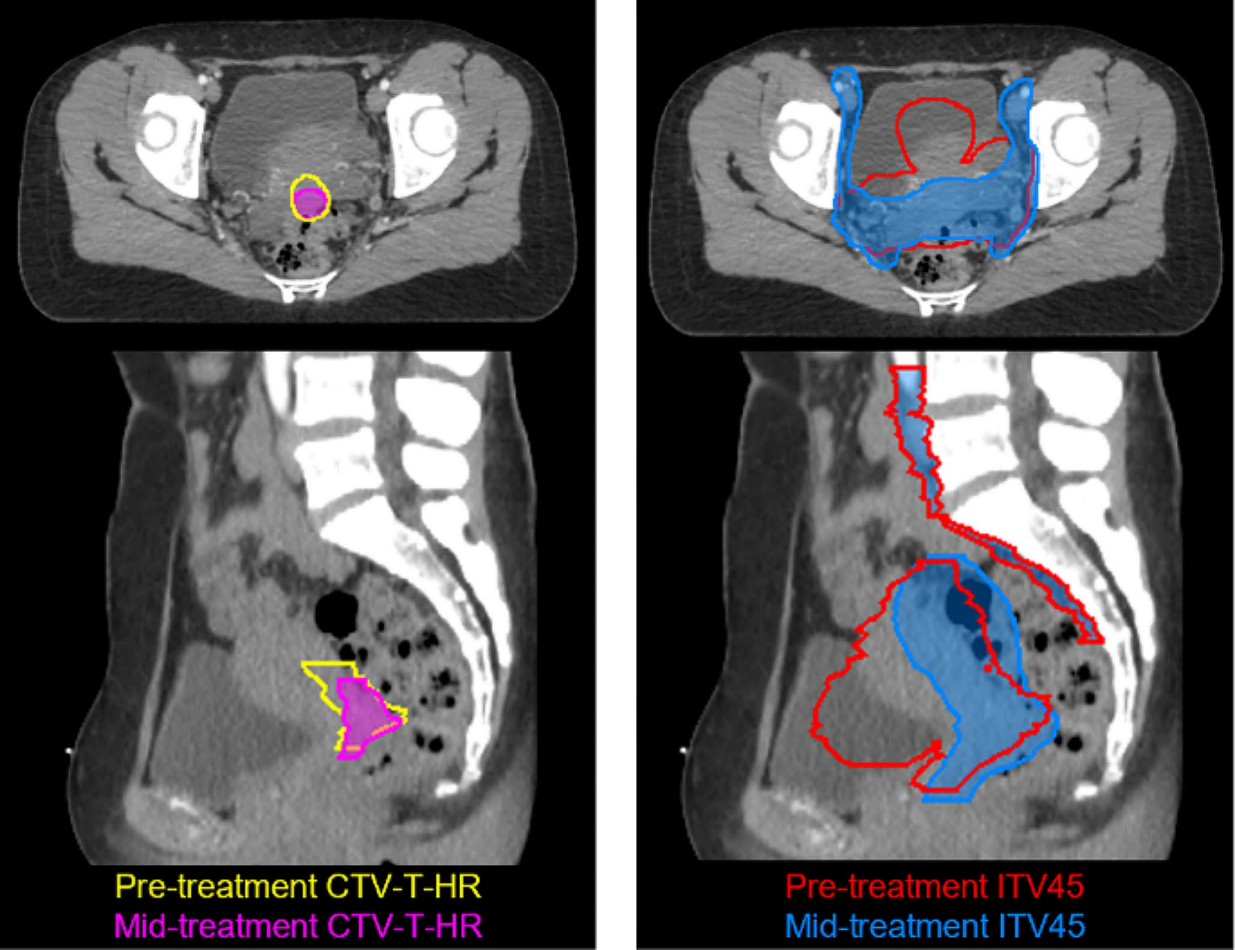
Example CT showing the changes between pre-treatment and mid-treatment contours (CTV-T-HR and ITV45)
